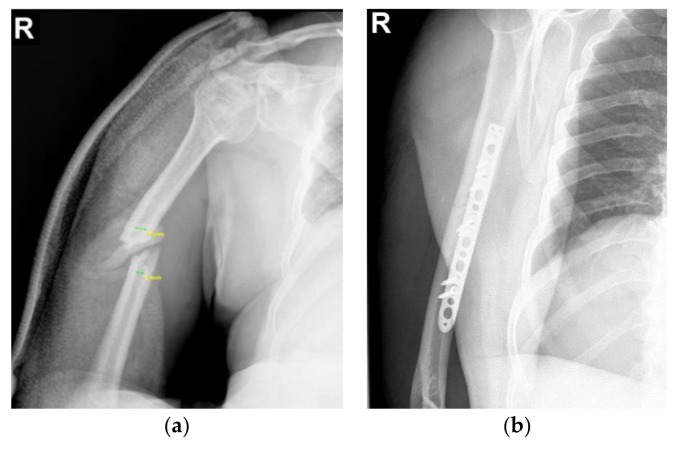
Clinical details: (a) X-ray comminuted fracture of midshaft humerus; (b) Post-operative X-ray with reduction and osteosynthesis with the locking compression plate (LCP).
